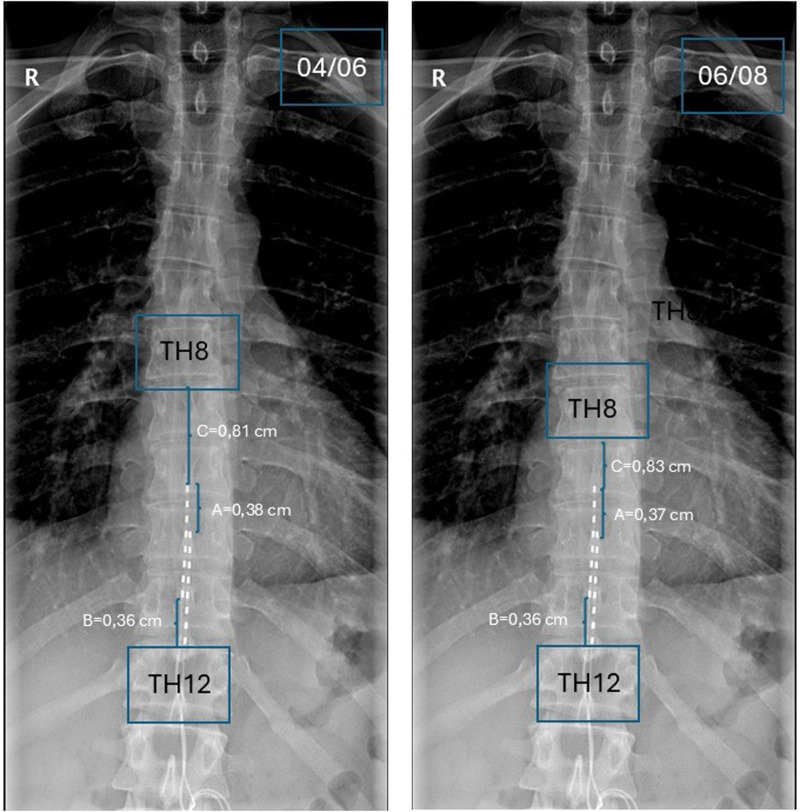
Comparative anteroposterior thoracic radiographs obtained on 04/06 (left) and 06/08 (right), showing TH8–TH12 levels and linear measurements (A, B, C) for assessment of vertebral alignment, with no significant interval change.
